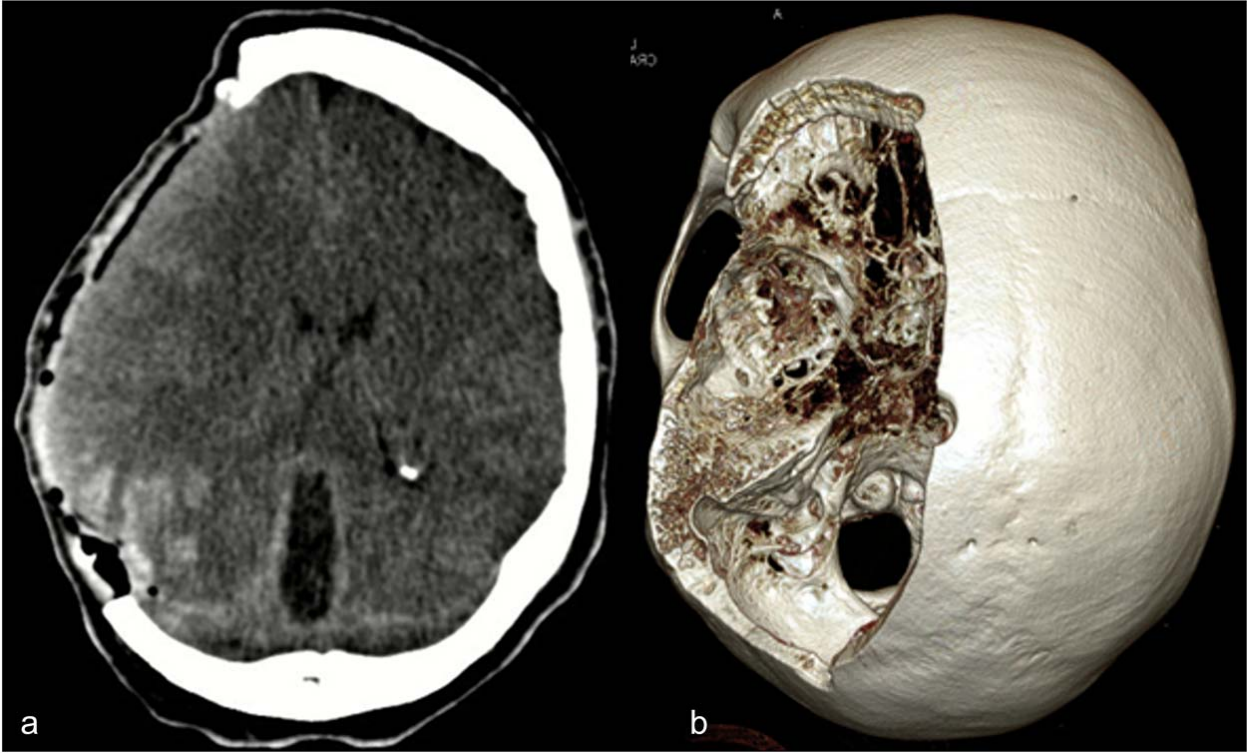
(a) Axial CT image after osteoclastic craniotomy and (b) in the 3D reconstruction representation of the large bone defect.
